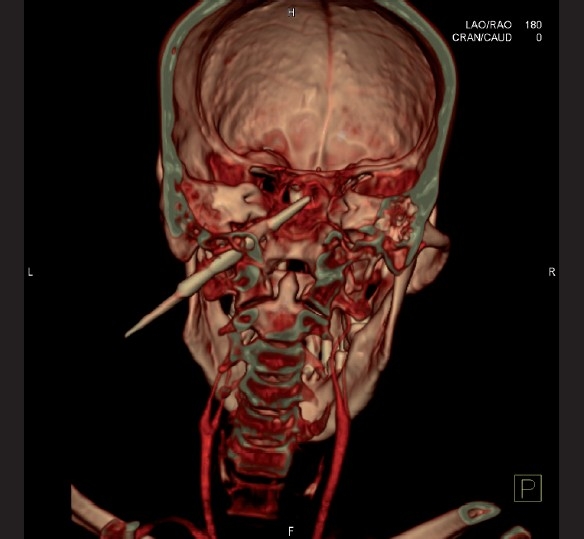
3D-computed tomography images showing the track of the arrowhead traversing just above the bifurcation of the common carotid
